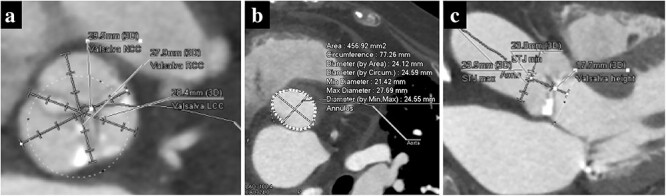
Preoperative computed tomography imaging showing a moderately calcified aortic annulus (a), an annulus area of 457 mm^2^ (b) and the short height of Valsalva (c).
